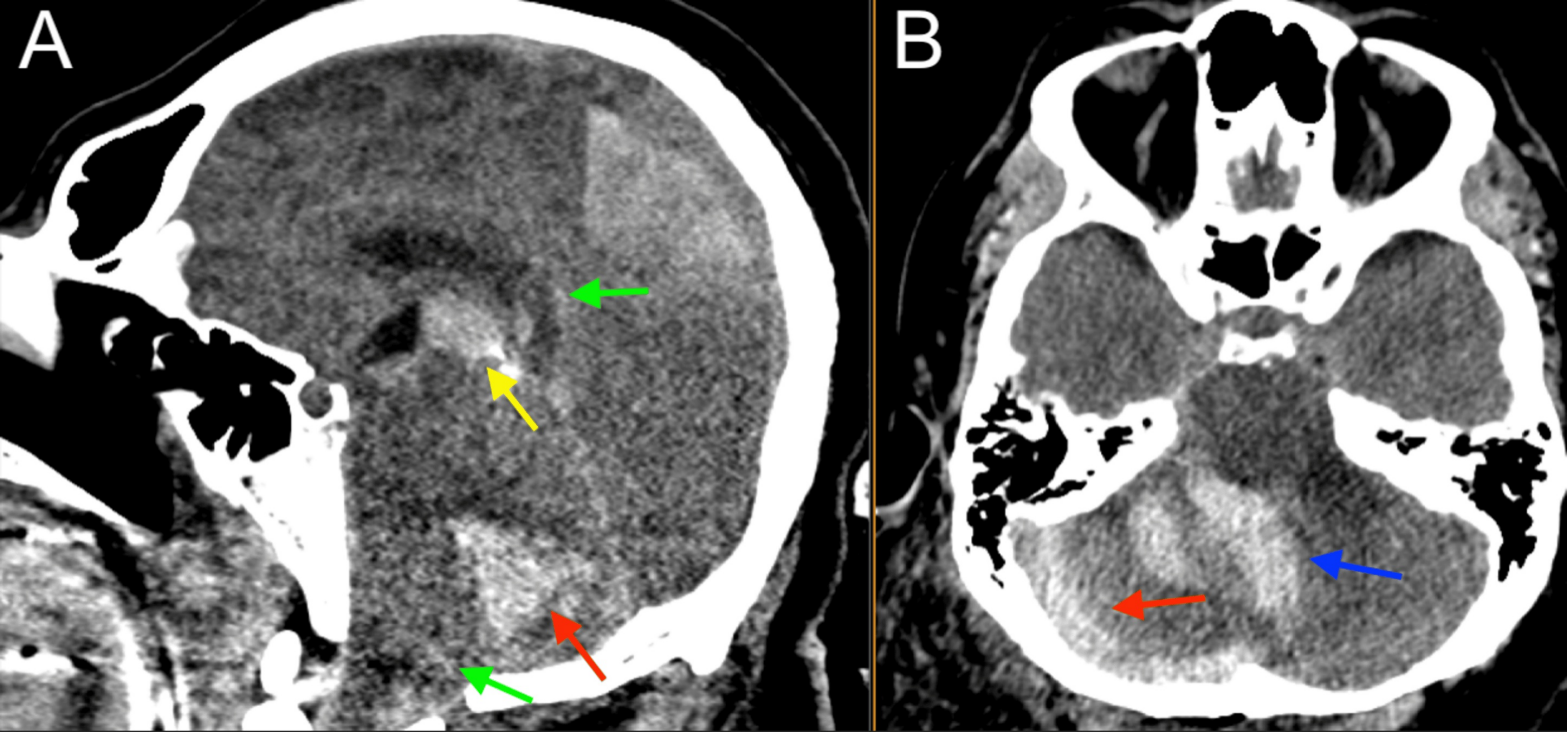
Head CT showing right cerebellar parenchymal hemorrhage (red), third ventricle intraventricular hemorrhage (yellow), fourth ventricle intraventricular hemorrhage (blue), foramen magnum crowding, and third ventricle upward herniation demonstrating posterior fossa fullness (green). (A) Sagittal view; (B) Axial view CT: computed tomography
